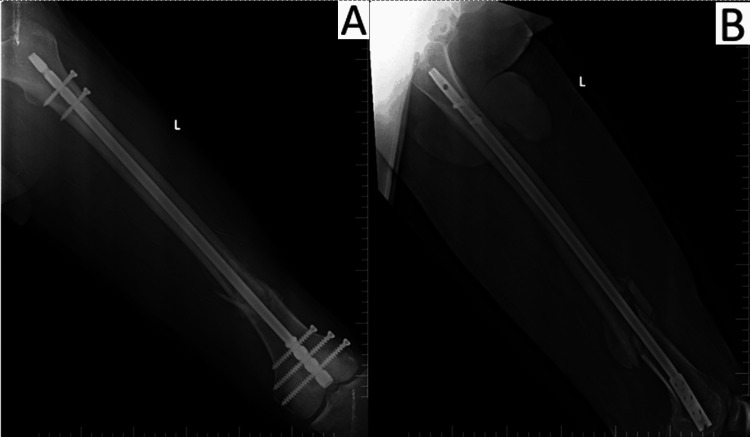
(A) Anteroposterior and (B) lateral X-ray views of retrograde intramedullary nailing showing nonunion (managed using bone marrow insertion)
